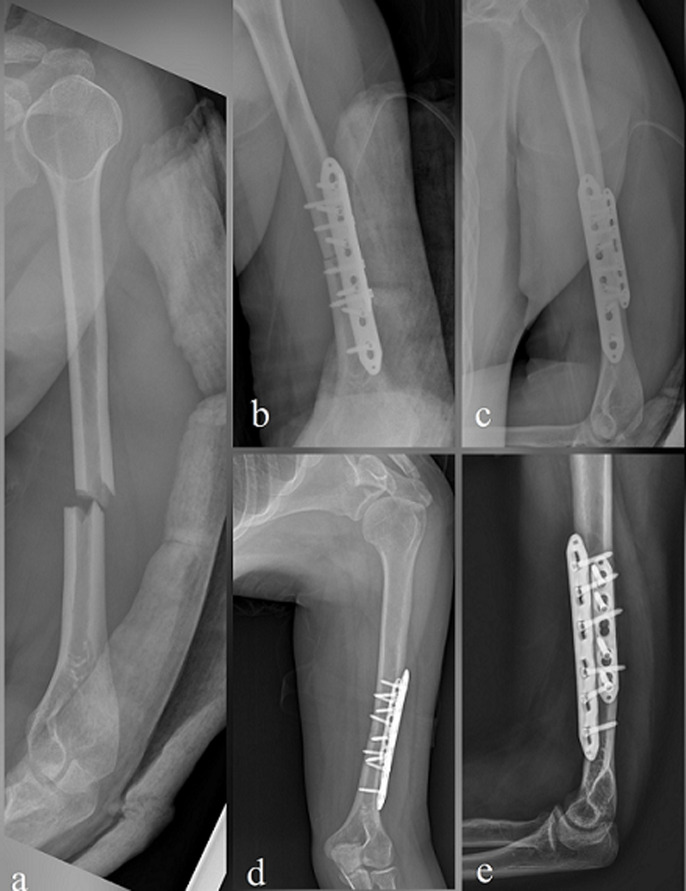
dual 3.5 mm LCP fixation; X-ray images of a 36-year-old woman with a left humeral shaft fracture as a result of a fall: (a) initial anteroposterior (AP) plane X-ray image; (b and c) early postoperative X-ray images; (d and e) X-ray images at 6^th^-month follow-up after surgery
